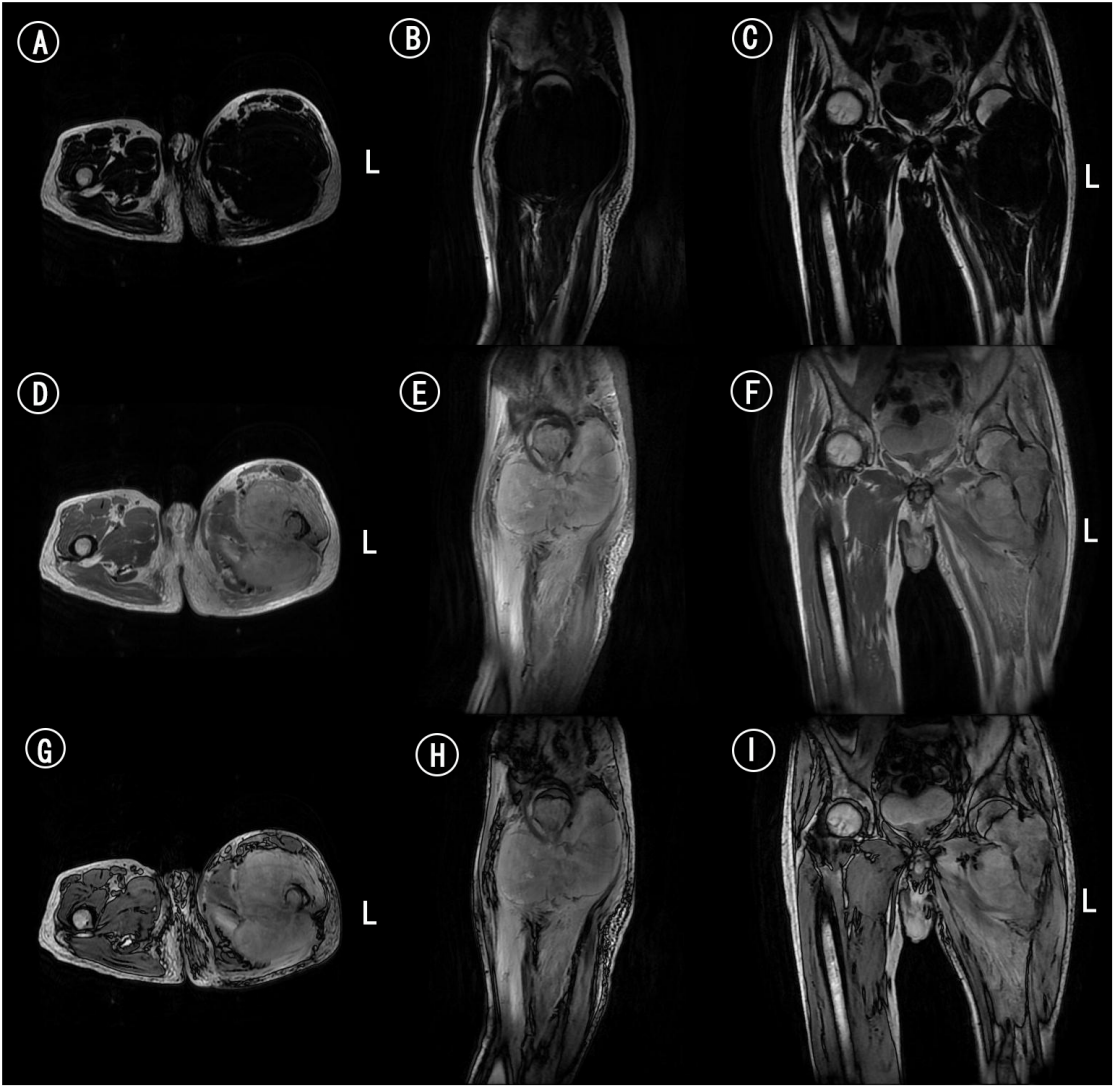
MRI of femur. (A) Axial MRI scan of femur in T1WI. (B) Sagittal MRI scan of femur in T1WI. (C) Coronal MRI scan of femur in T1WI. (D) Axial MRI scan of femur in T2WI. (E) Sagittal MRI scan of femur in T2WI. (F) Coronal MRI scan of femur in T2WI. (G) Axial MRI enhanced scan of femur. (H) Sagittal MRI enhanced scan of femur. (I) Coronal MRI enhanced scan of femur.
